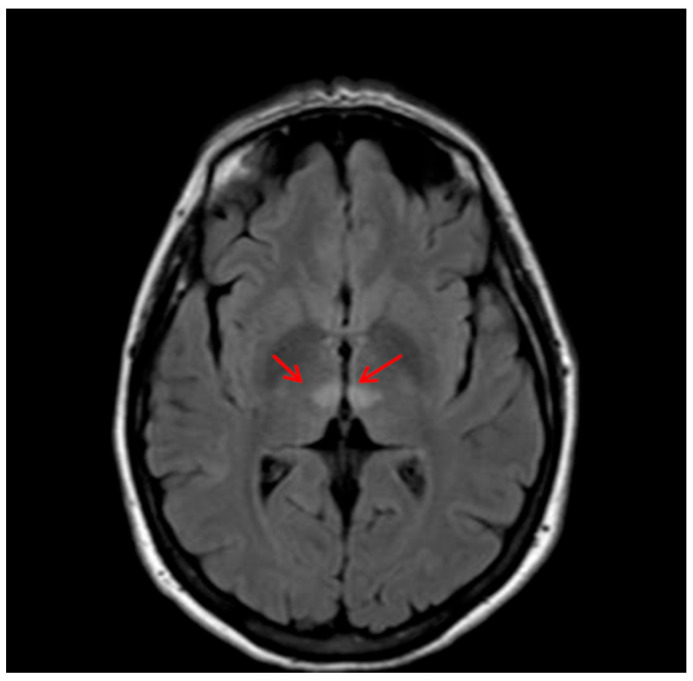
Cerebral MRI with the appearance of bilateral thalamic ischemia—Percheron artery. T2 sequence.
